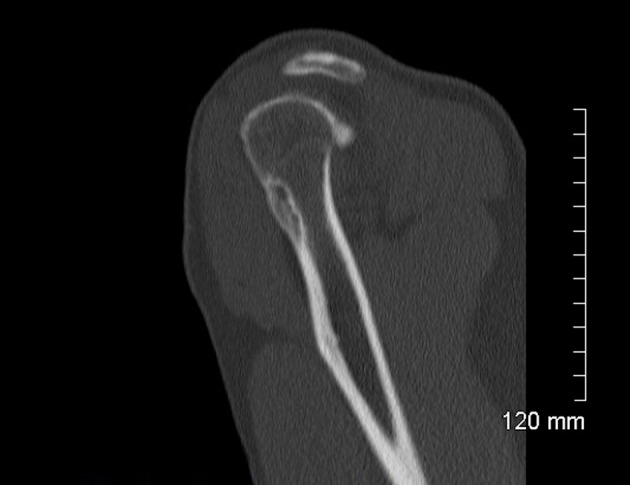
CT hypodense lacunar formation on the front cortical bone.
